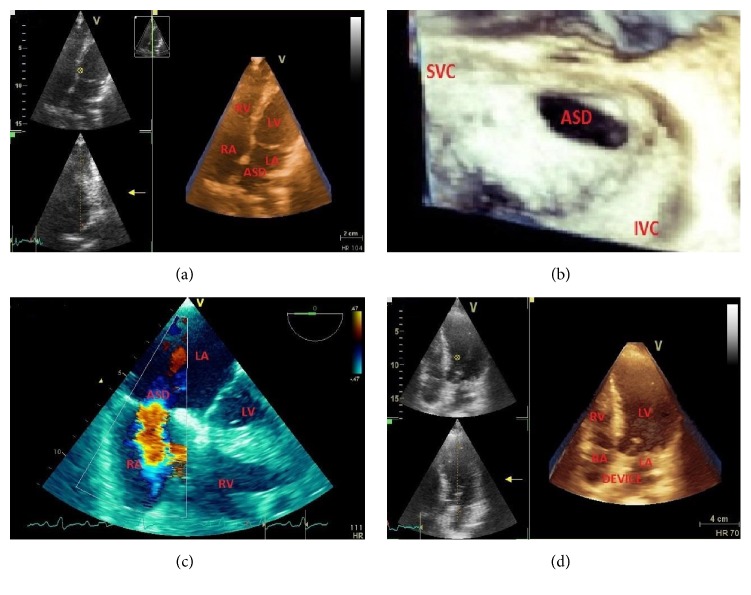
A 32-year-old female patient with ostium secundum atrial septal defect (ASD) and a history of ischaemic stroke. Patient had atrial device implantation. (a) Three-dimensional apical 4-chamber view, ASD. (b) Transesophageal three-dimensional longitudinal view, ASD shape. (c) Transesophageal 4-chamber view, left-to-right shunt by color Doppler. (d) Postdevice three-dimensional apical 4-chamber view showing absence of shunt flow. IVC = inferior vena cava; LA = left atrium; LV = left ventricle; RA = right atrium; RV = right ventricle; SVC = superior vena cava.
